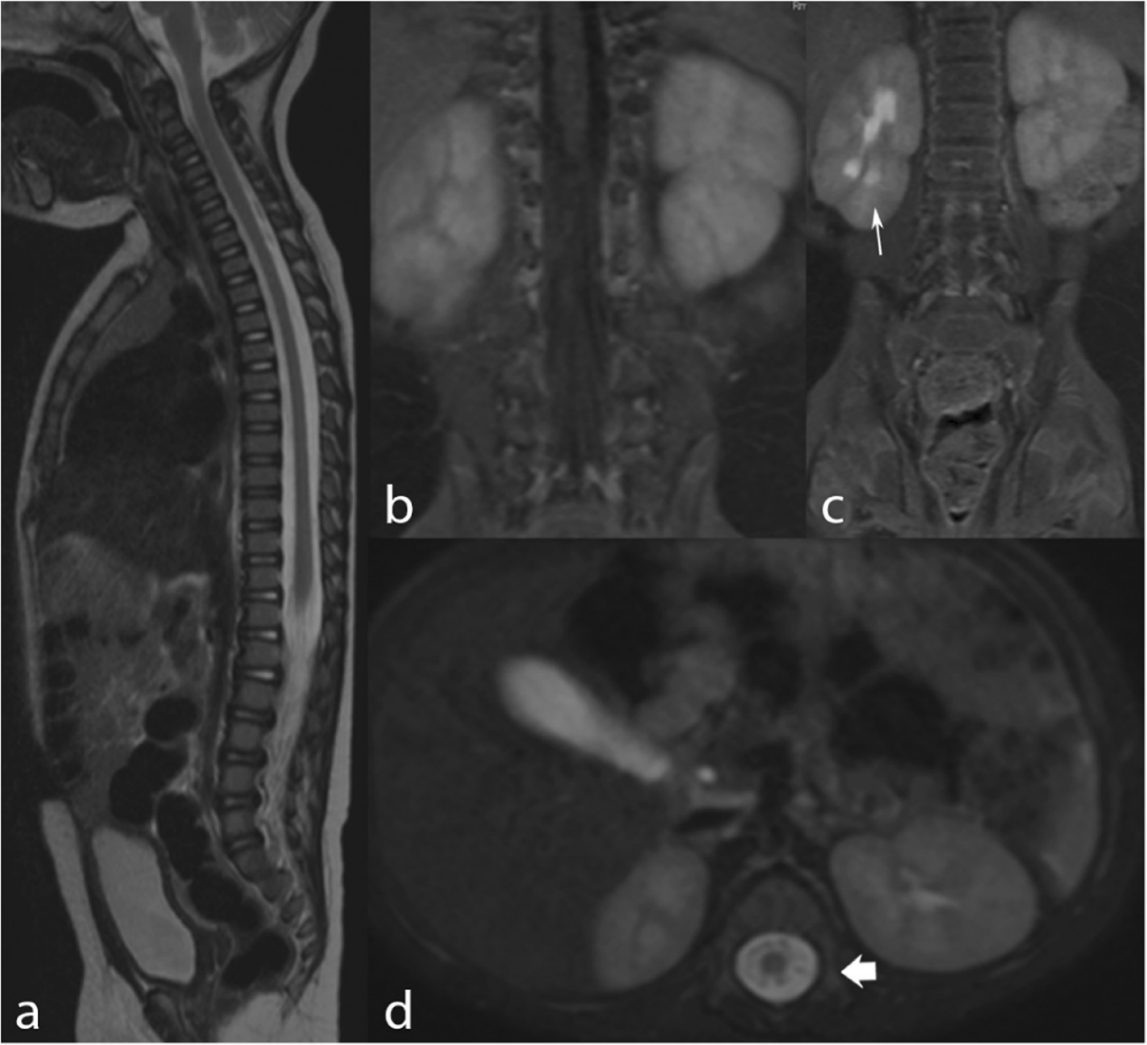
MRI after conclusion of chemotherapy. Three planar T2WI (a, c, d) and coronal T1WI (b). The exam shows complete regression of the tumor at both intra- and extraspinal compartments. Mild pyelectasia was detected in the right kidney
